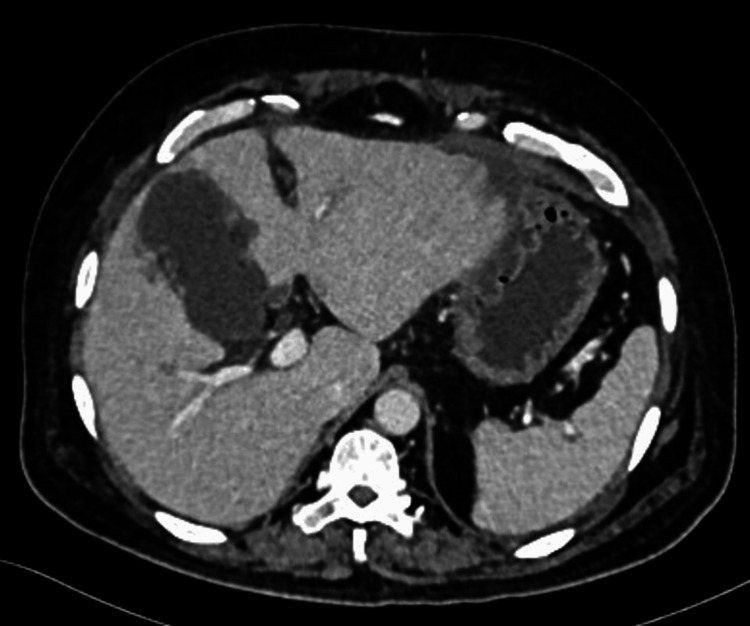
CECT abdomen showing pathological gallbladder having distended lumen with irregular walls which appear dehiscent at places, multiple loculated fluid collections in the pericholecystic and perihepatic subcapsular regions CECT: contrast-enhanced computed tomography
